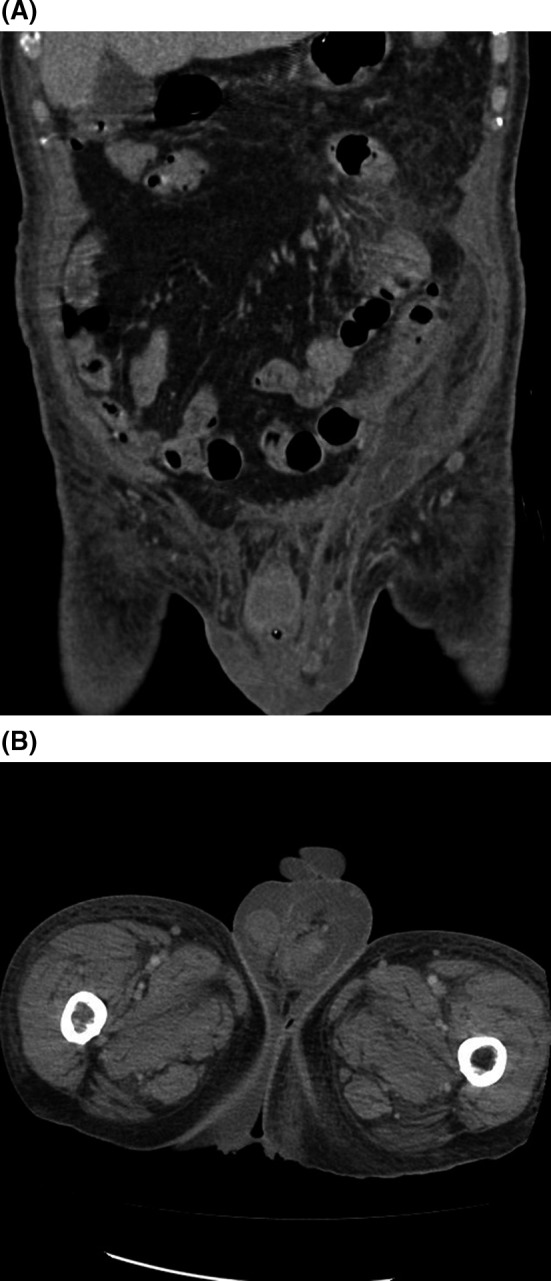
Coronal CT (A) and axial CT (B) show fluid collection in the retroperitoneum, flowing into the left scrotum via the left inguinal canal
